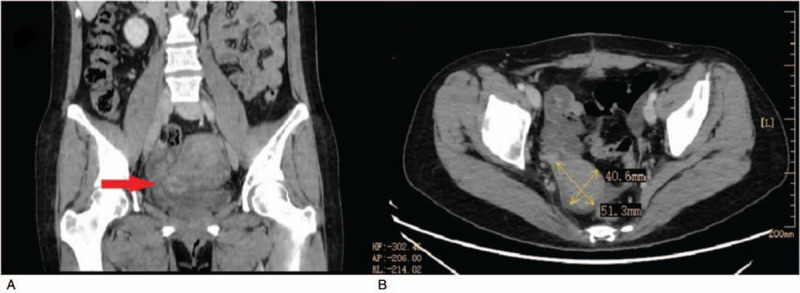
computed tomography scans of case 14. (A) preoperation: a mass of slightly higher density shadows was seen on the right side of the uterus, and the contrast-enhanced scan showed nodular and ring enhancements (arrow). (B) Forty-three months after surgery: A slightly hypodense solid soft masses, which was slightly enhanced in enhancement scan computed tomography scans, was found in the right posterior part of the pelvic cavity.
